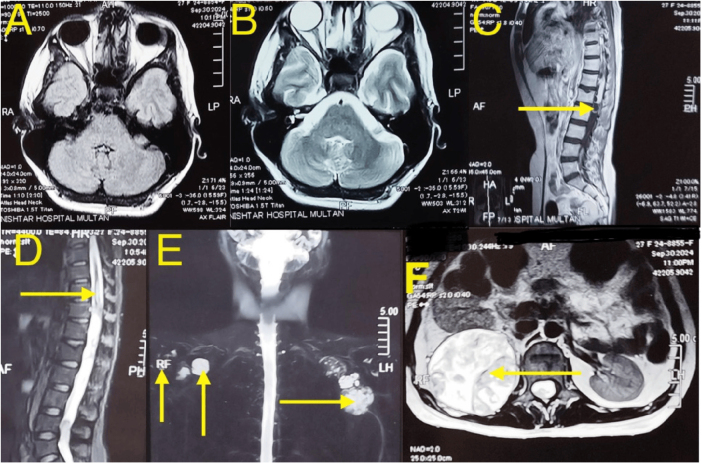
MRI brain plain, axial sections FLAIR sequence (A) and T2W sequence (B), show no abnormal MR signal intensity lesion (vestibular schwannoma) at bilateral cerebello-pontine angles. Post-contrast MRI thoracolumbar spine, Sagittal section T1W sequence with contrast (C), shows heterogeneously enhancing solid cystic intramedullary extradural lesion seen opposite D11 to L5 levels. MRI thoracolumbar spine, Sagittal section T1W with fat suppression (D), shows hyperintense abnormal MR signals returning from cord opposite D10–D11 vertebral levels. Post-contrast MRI spine, coronal section, myelogram (E), shows solid enhancing lesions along bilateral anterior chest and medical aspect of right upper arm. Post-contrast MRI spine, T1W sequence with contrast, axial section (F), showing solid enhancing lesion in right lumbar region.
